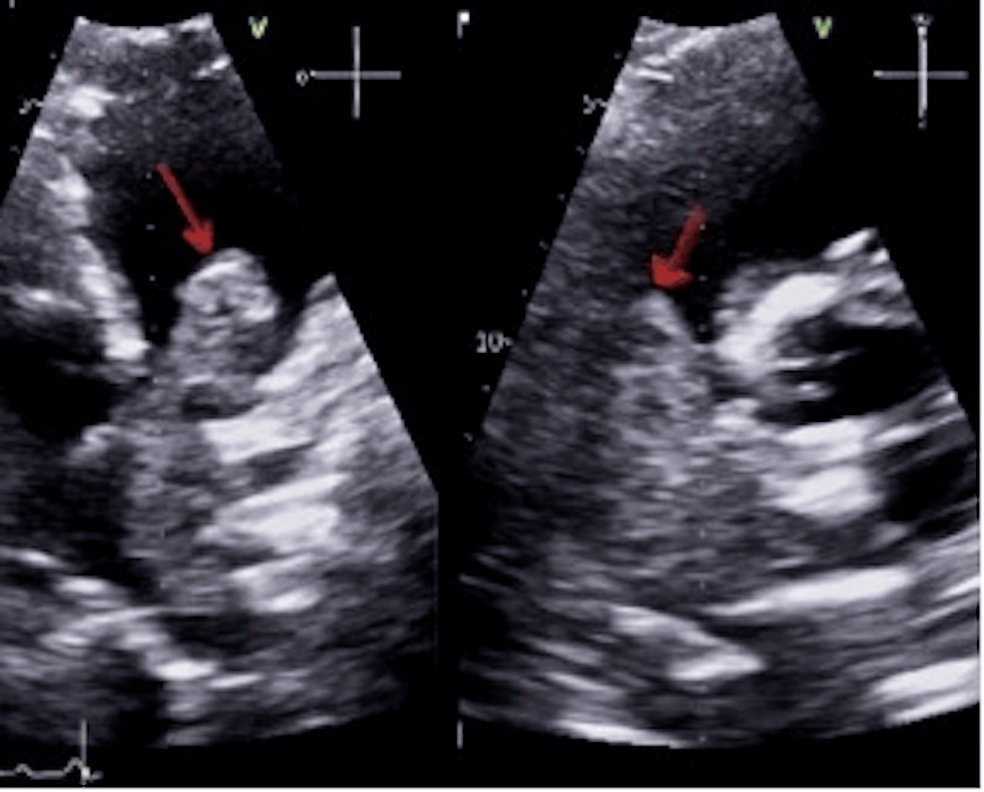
The echocardiography study showed a large hypoechoic mass inside the pulmonary artery extending to the right ventricle lumen (red arrows)
